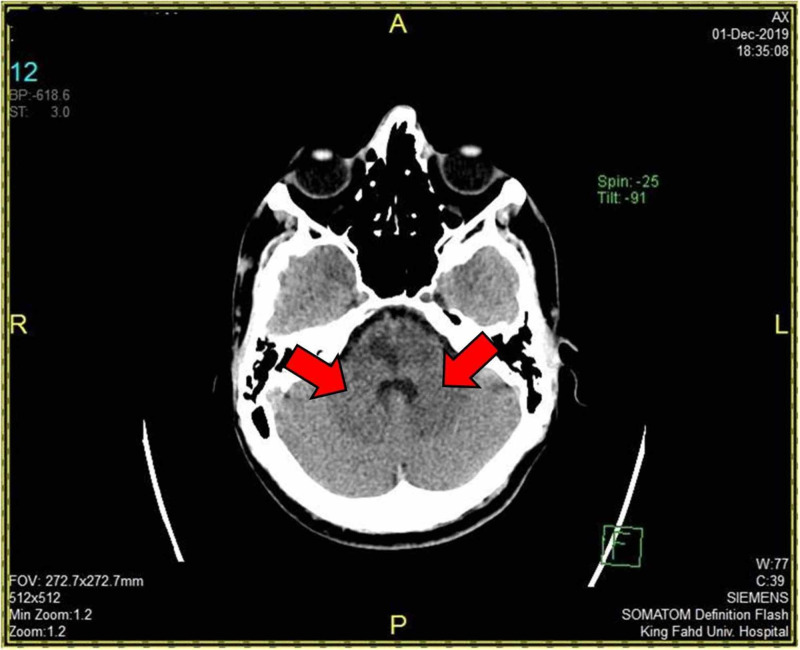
Computed tomography (CT) of the brain Axial view of the brain reveals pontine hypodensity.
